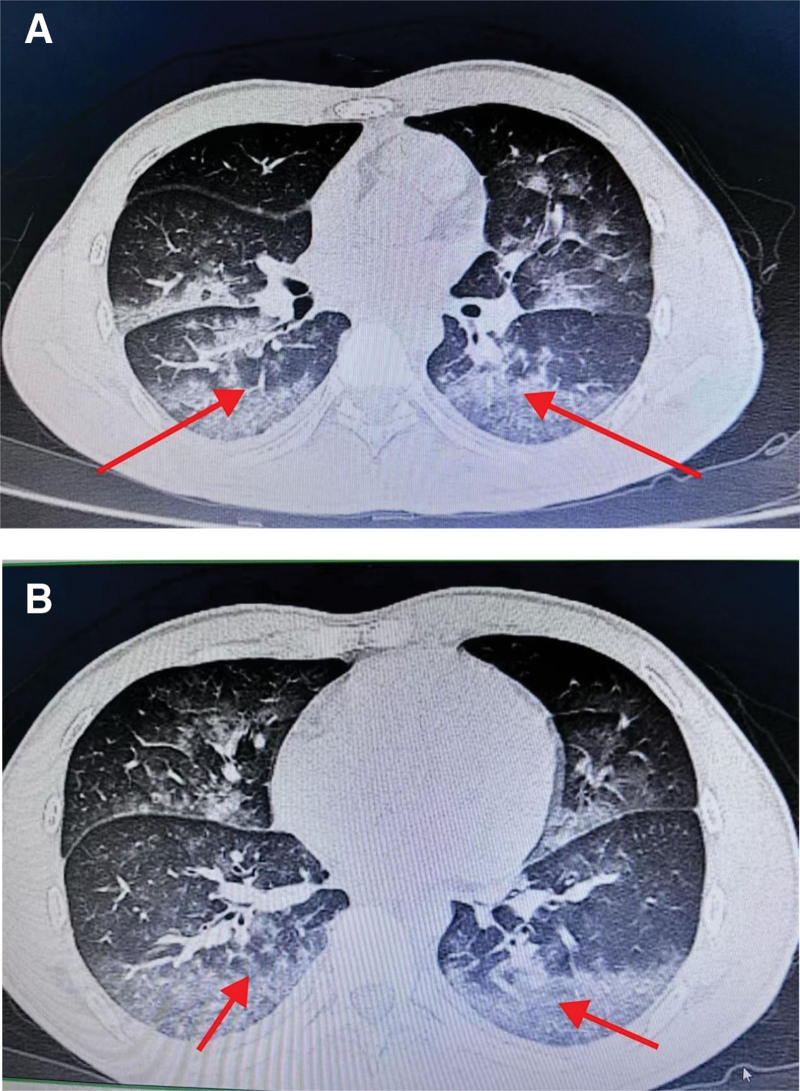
(B1, B2) Postoperative chest CT scans of the same area. CT = computed tomography.
